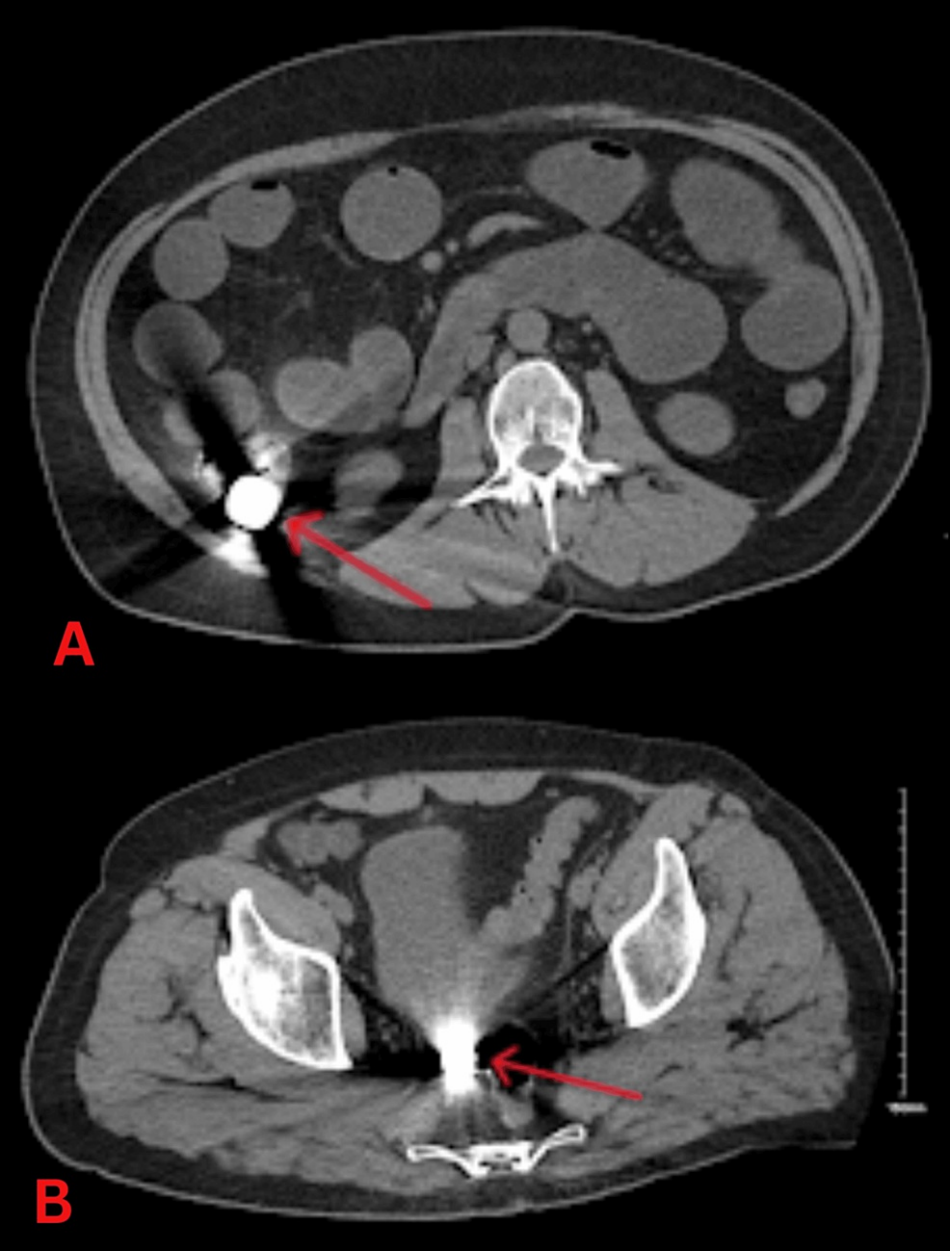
CT scan revealing multiple foreign bodies in the cecum (A) and small bowel (B) (arrows) CT: computed tomography
